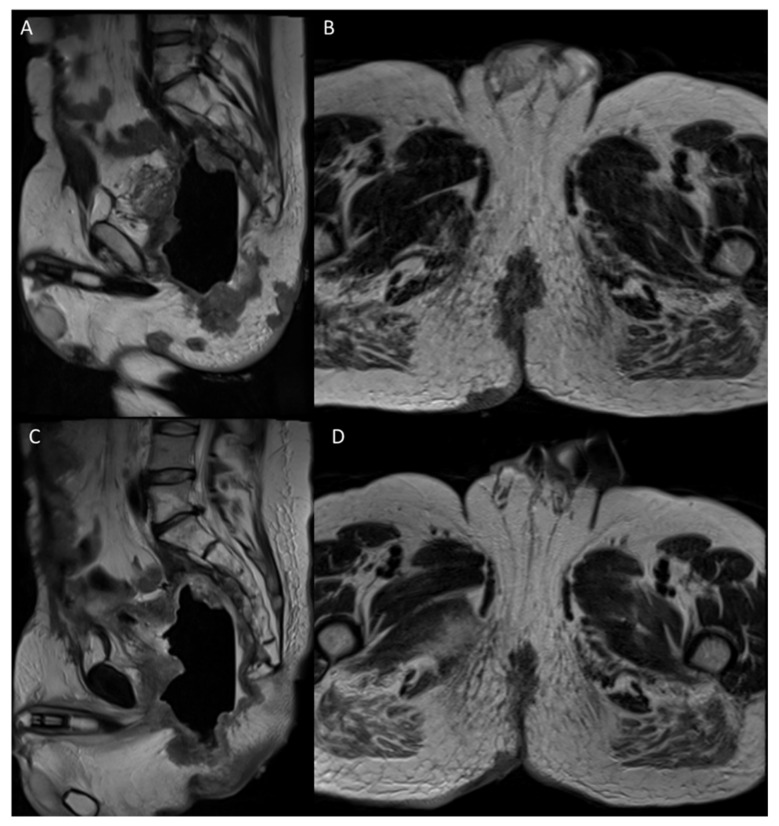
MR images (A,B) before treatment of third case and 6 months after ECT treatment (C,D).
